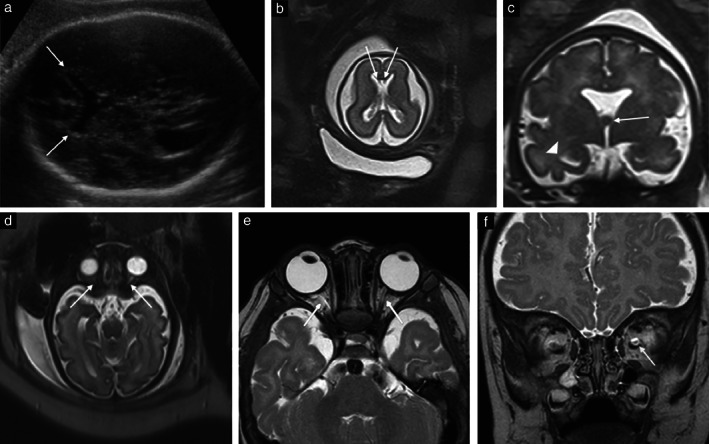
Prenatal and postnatal imaging in fetuses with isolated septal agenesis that had postnatally confirmed septo‐optic dysplasia. (a) Axial ultrasound image at 31 + 1 weeks' gestation, demonstrating fused anterior horns, absence of cavum septi pellucidi and squared anterior horns (arrows). (b) T2‐weighted axial magnetic resonance (MR) image at 23 + 4 weeks, demonstrating fused anterior horns and septal remnants (arrows). (c) T2‐weighted coronal MR image at 34 + 0 weeks, demonstrating fused forniceal columns (arrow) and dysplastic temporal horns (arrowhead). (d) T2‐weighted axial MR image at 33 + 6 weeks, demonstrating bilaterally hypoplastic optic nerves (arrows). (e) T2‐weighted axial MR image at 4 months of age, demonstrating hypoplastic extra‐orbital optic nerves (arrows). (f) T2‐weighted coronal MR image at 4 months of age, demonstrating hypoplastic nerve within fluid‐filled optic nerve sheath (arrow).
